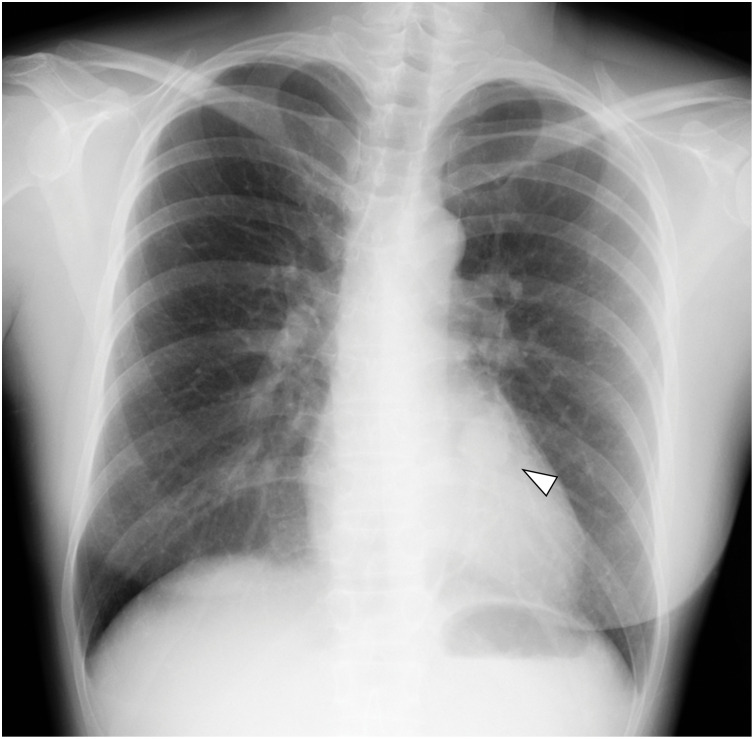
Preoperative chest radiograph showing the tumor (white arrowhead).
